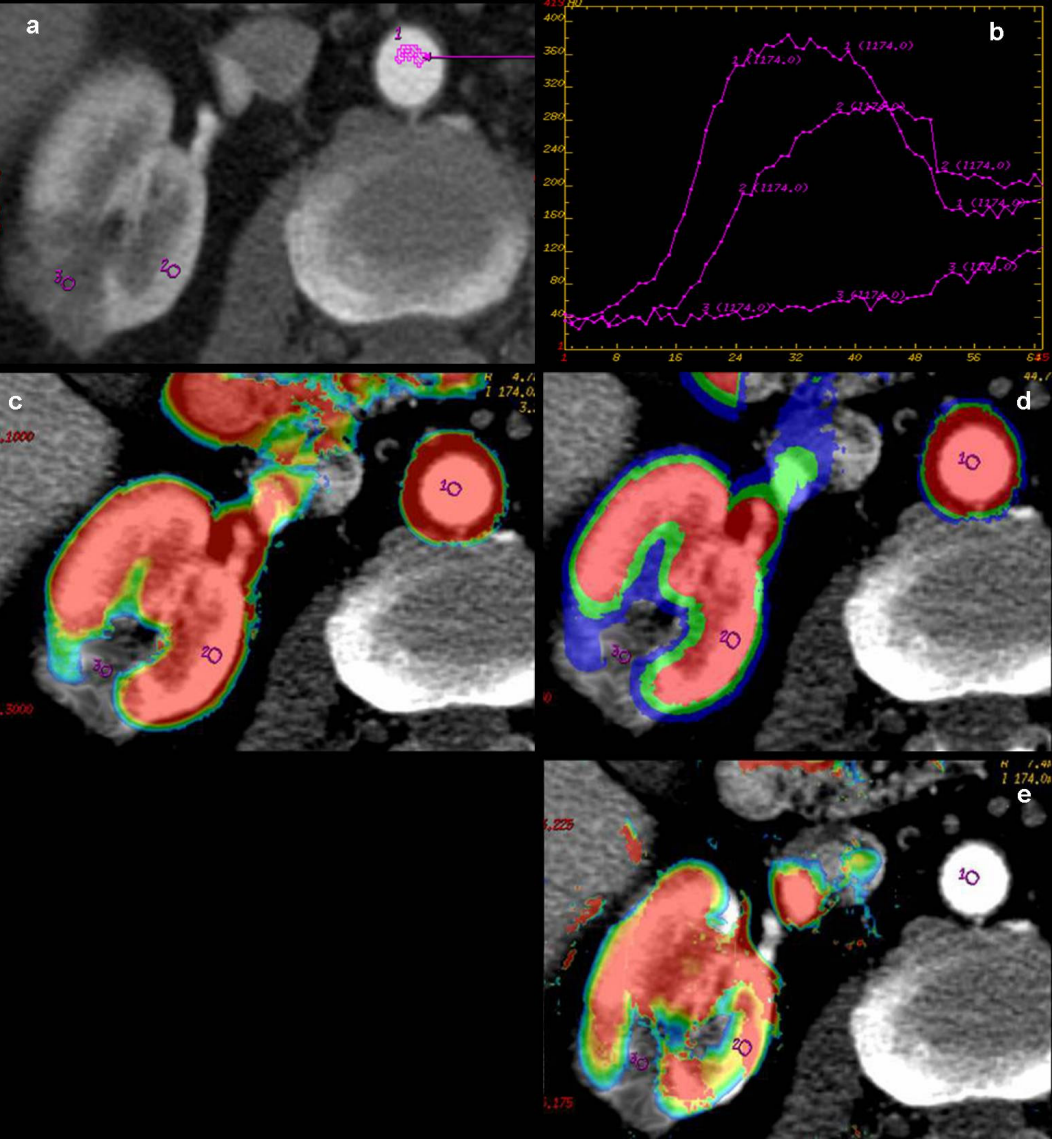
Cryoablated Renal Cell Carcinoma (RCC) in the right kidney of a 47 years-old patient. a) Perfusional CT scan shows three regions of interest, selected on abdominal aorta (ROI 1), normal ipsilateral renal cortex (ROI 2), cryoablated tumor area (ROI 3). b) The corresponding time-density curves show contrast enhancement kinetic with typical pattern at responsive cryoablated tumor area (curve 3: slower initial enhancement, decreased amplitude, slower wash-out) compared to abdominal aorta (curve 1) and ipsilateral normal renal cortex (curve 2). Blood colour maps (c, Blood Volume, BV; d, Blood Flow, BF; e, Permeability - Surface Area Product, PS) at the same levels, show the high arterial (ROI 1) and parenchymal (ROI 2) perfusion parameters with no colour encoding in successfully cryoablated area (ROI 3).
